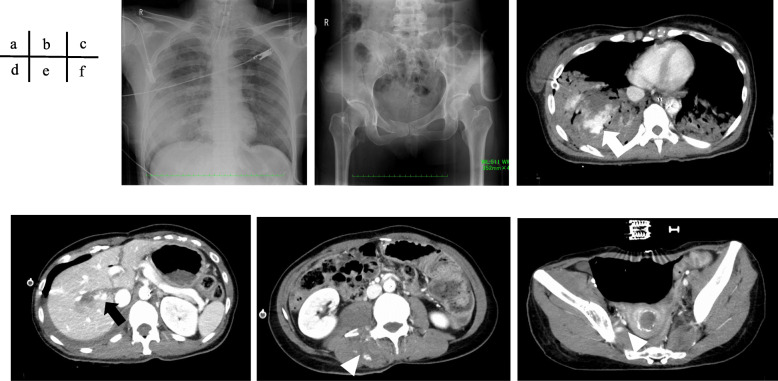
X-ray and computed tomography findings on admission. a Chest X-ray showing heavy contusion and hemopneumothorax in the right lung. b Pelvis X-ray showed disruption of bilateral sacroiliac articulation and fractures of bilateral pubic bone. c A chest computed tomography scan revealed massive lung contusion with major active extravasation (*white arrow*) in the lower lobe of the right lung and moderate lung contusion in the lower lobe of the left lung. d Abdominal computed tomography scan revealed liver injuries with extravasation (*black arrow*). e, f Pelvic computed tomography showed massive hematoma with extravasation (*white arrow head*) in erector spinae muscle and fractures of transverse process of lumbar vertebrae (e) and multiple pelvic fractures involving moderate hematoma with extravasation of contrast media in retroperitoneal pelvic space (f)
